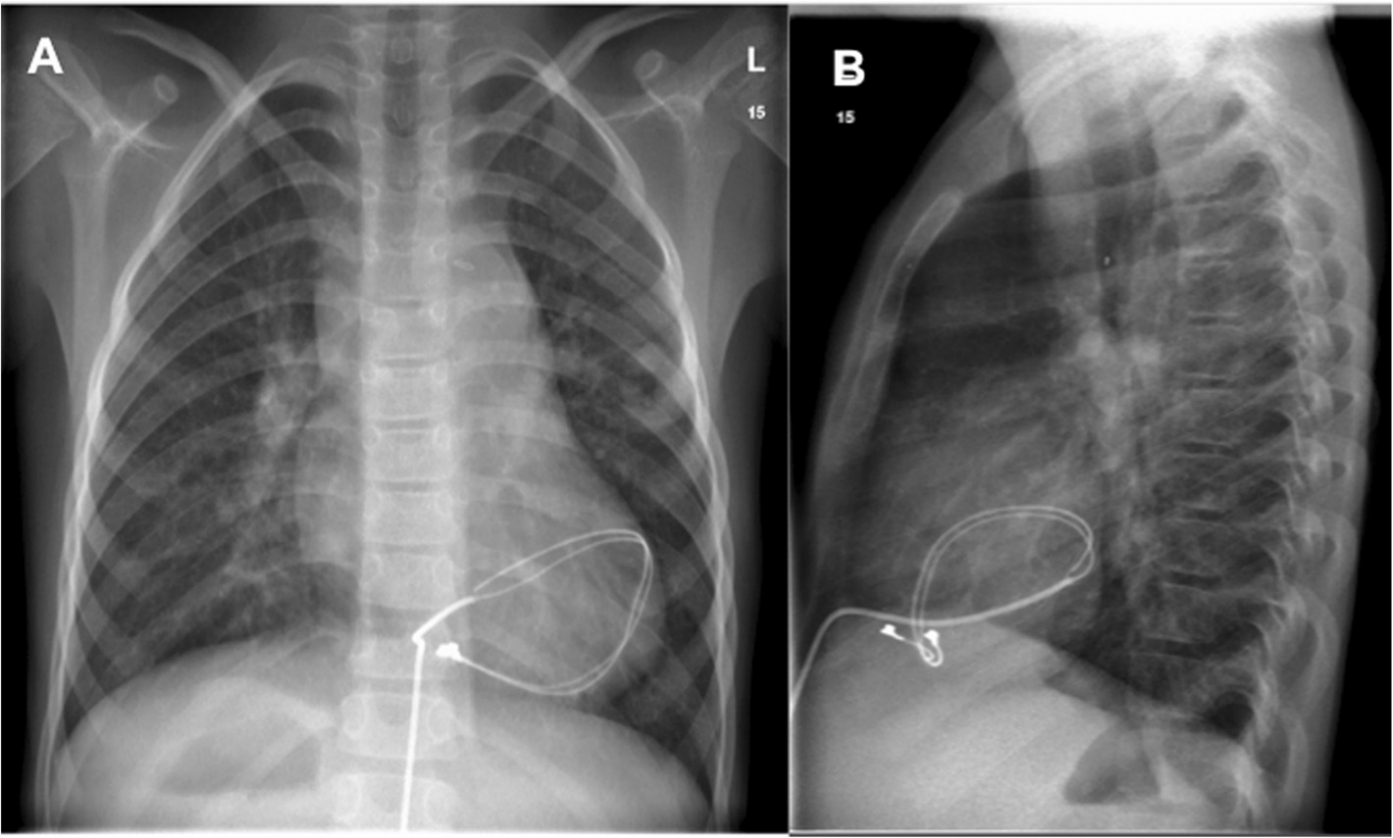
Shows the chest x-ray of the second patient with cardiac strangulation by an epicardial pacemaker lead. (For details read Results). A: Chest x-ray a.p. projection. B: Lateral projection showing the abnormal course of the RV lead, which forms a loop around the left ventricle. Note the course of the lead within the border of the heart shadow in panel B.
